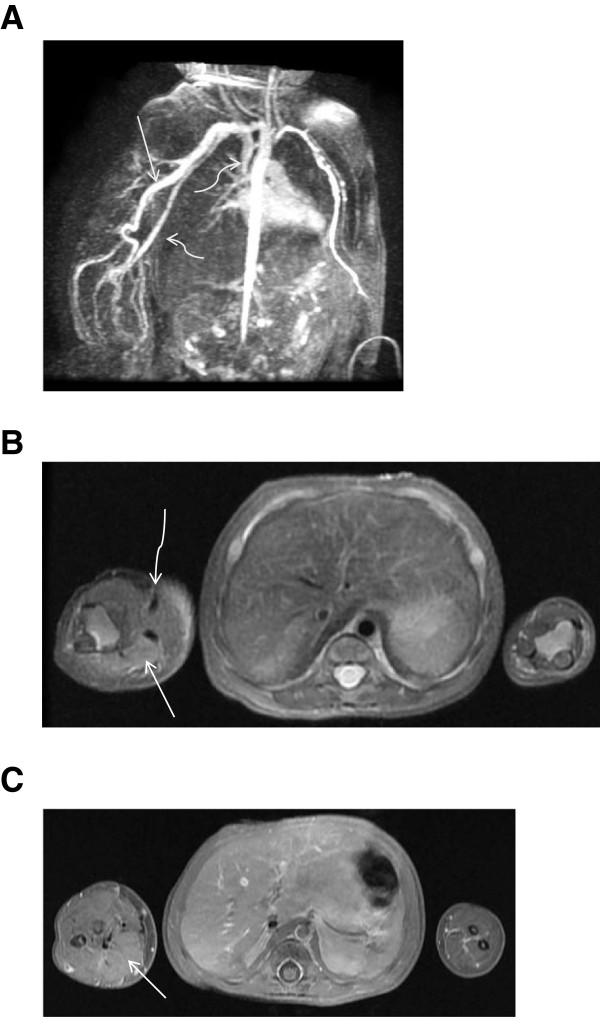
MRI 3A)Time-resolved MR angiogram shows near simultaneous opacification of a markedly dilated right upper extremity artery(arrow)and vein(curved arrows). 3B) T2 weighted axial sequence shows increased signal and enlargement of right upper extremity soft tissues (arrow) and large vascular flow voids (curved arrow). 3C) T1 fat saturated post-gadolinium axial sequence suggests enhancement of the musculature in the right forearm (arrow) compared with the left forearm.
